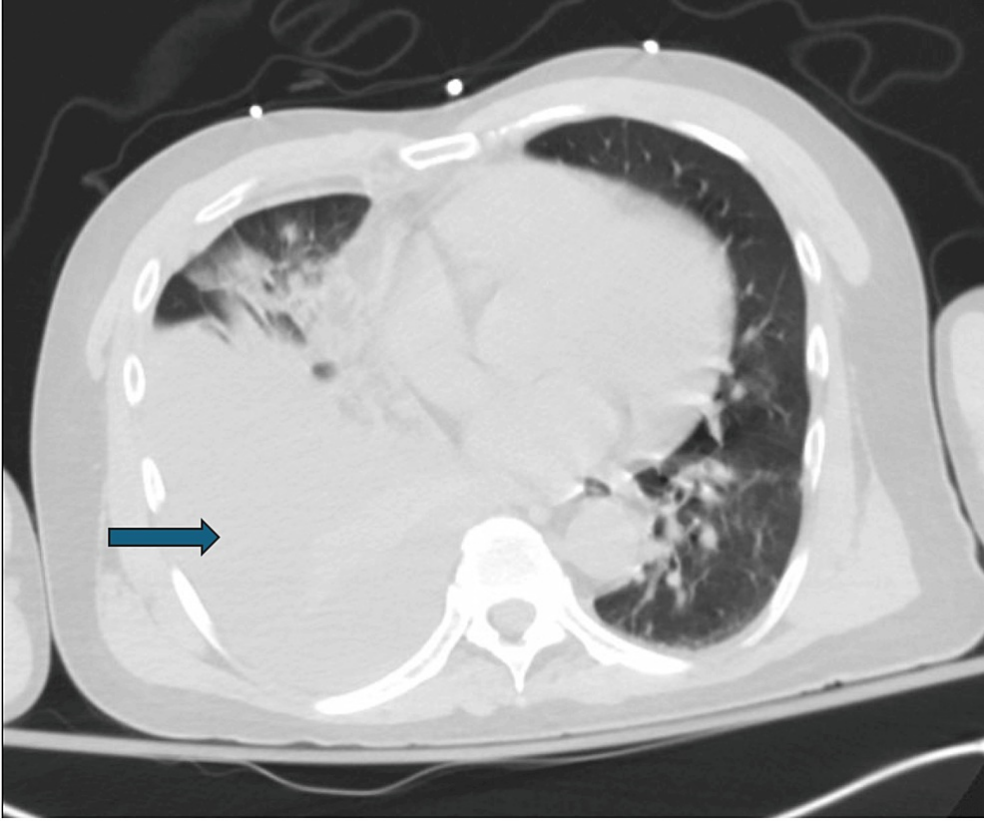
The initial CT scan of the chest reveals a moderately-sized right-sided pleural effusion.
